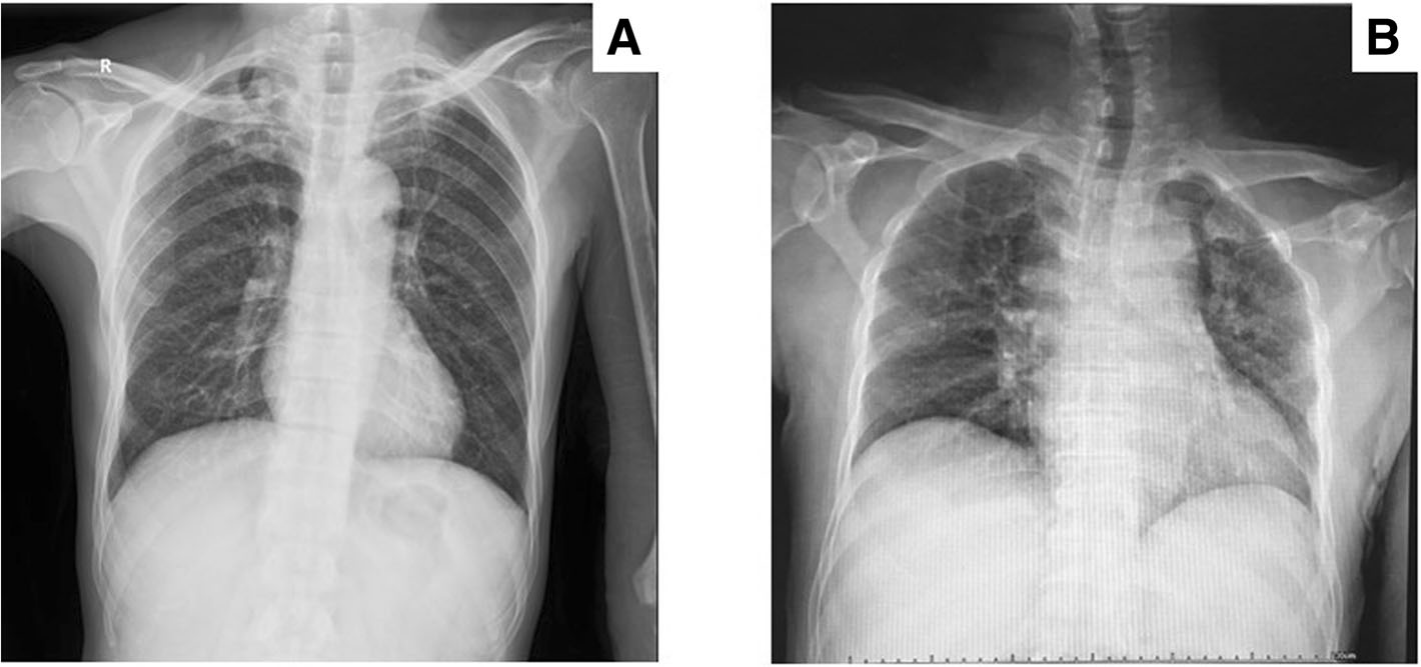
X-ray of pulmonary tuberculosis. a Moderate infection; b Severe infection
